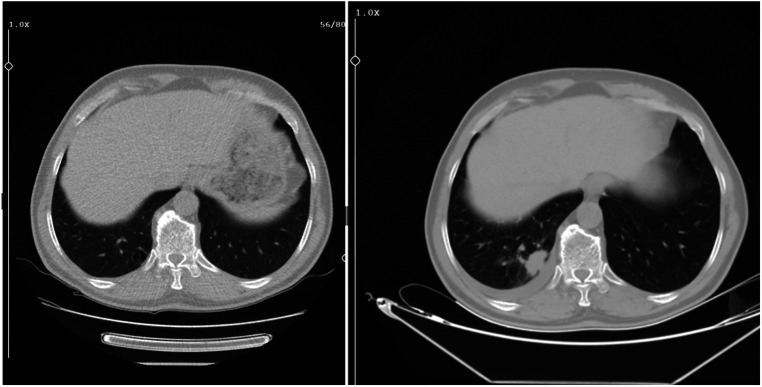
Thorax computed tomography taken 2 years prior (left) and at the time of diagnosis (right).
